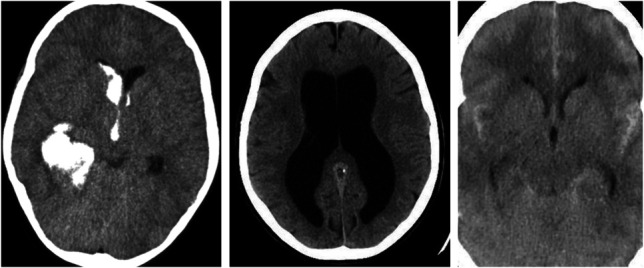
Case 1 (left), Case 2 (middle), and Case 3 (right) depicting intraventricular hemorrhage, hydrocephalus, and narrow slit-like ventricles, respectively. Respondents were asked to select which case that they would find image guidance helpful for and were presented with these single axial computed tomography slices alone.
